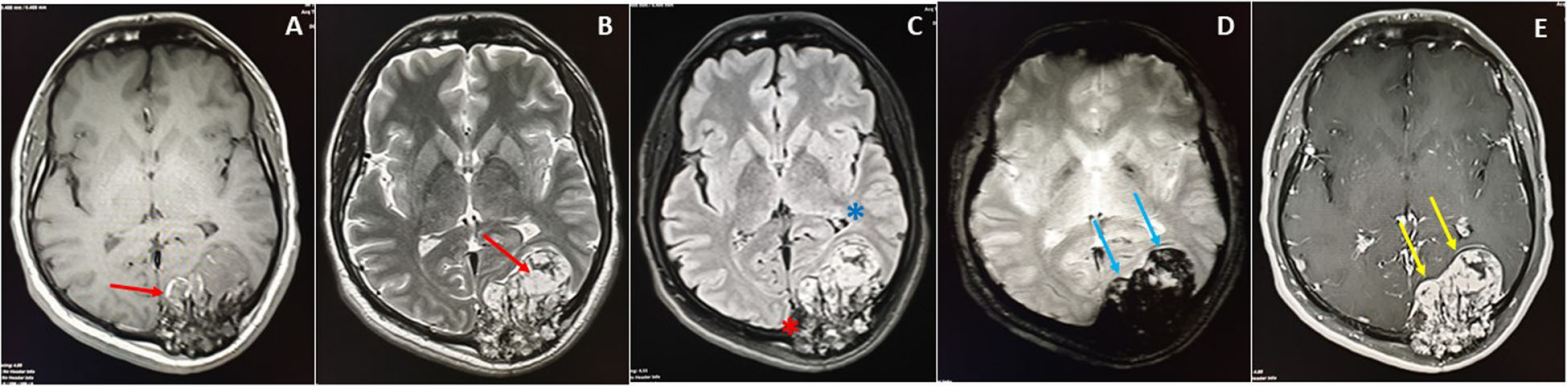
MRI images T1WI (a), T2WI (b), FLAIR (c), Hemo (d), and post contrast (e) the left occipital lesion showed hyperintensities on T1WI/T2WI of fat and vascular content respectively (Red arrow), and blooming effect (Blue arrows) on Hemo images of hemorrhagic content, and intense post-contrast enhancement (Yellow arrows). It compressed the adjacent occipital lobe without edema, deviating anteriorly the occipital horn (Blue asterisk) of the left lateral ventricle, and obstructing the posterior aspect of the superior sagittal sinus (Red asterisk)
